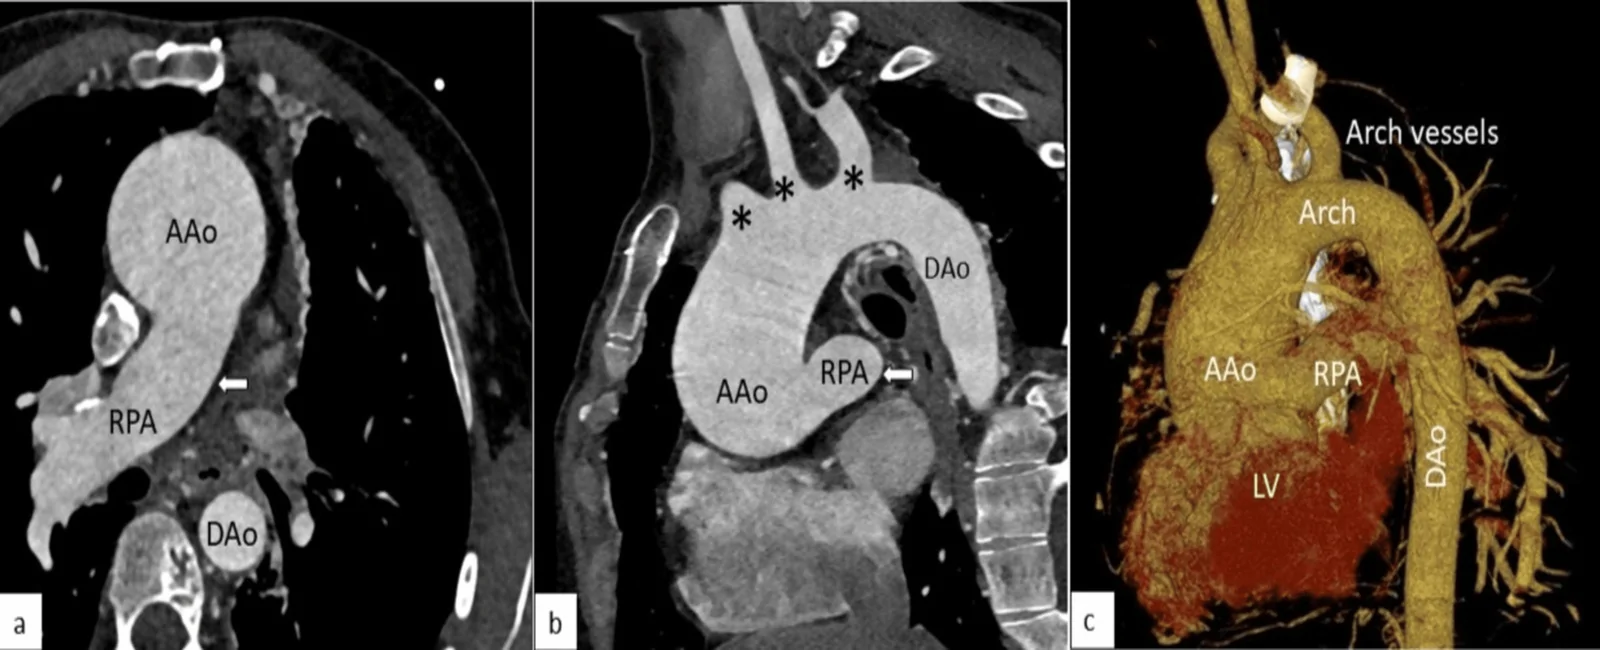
Cardiac CT showing right Hemitruncus (a and b) Multislice Cardiac CT (a) Axial and (b) Sagittal (reformatted oblique) images showing anomalous origin of RPA from ascending aorta (white arrow); (b) Sagittal (reformatted oblique) images showing anomalous origin of RPA from ascending aorta (white arrow) with normal origin of the arch vessels (asterisks). (c) Volumetric reconstruction image showing the origin of the RPA from the Ascending aorta. RPA- right pulmonary artery, AAo- Ascending Aorta, DAo- Descending aorta, LV- Left ventricle.
